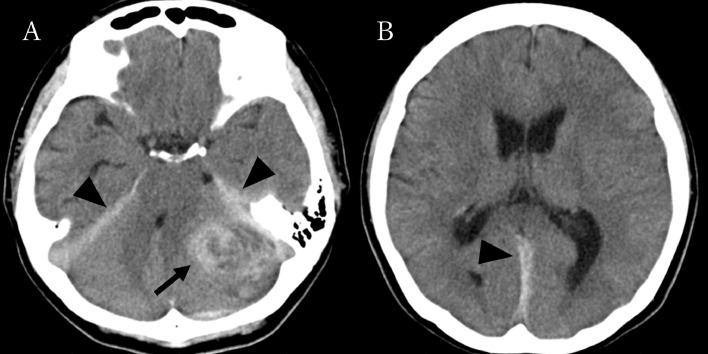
Precontrast computed tomography scans of the head showing (A) a hemorrhagic mass lesion in the left cerebellar hemisphere (arrow) and acute subdural hematoma extending along the cerebellar tentorium (arrowheads), and (B) slightly enlarged ventricles with acute subdural hematoma extending along the falx cerebri (arrowhead).
